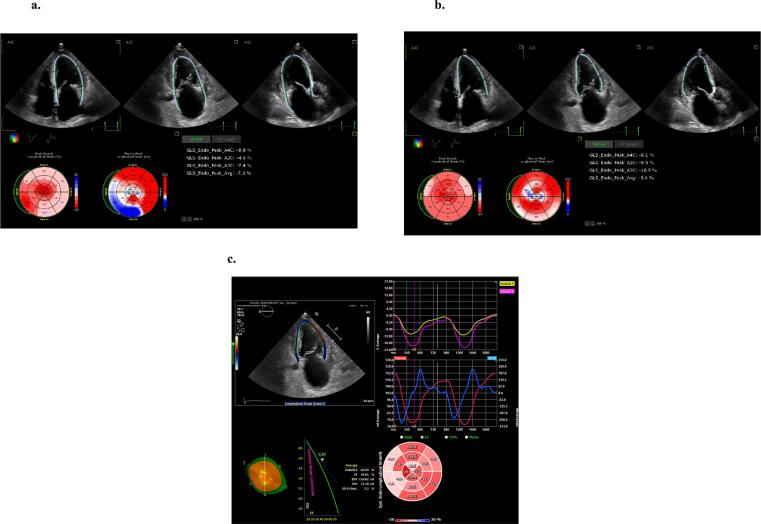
Representative case of full-automatic (a), semi-automatic (b) and manual (c) assessed LS. This was representative case of poor automatic assessment by LS. Automated trace lines were not drawn along the true endocardial border (a). Therefore, investigator manually corrected borders for semi-automatic assessment (b).2.Semi-automatic assessment: after the automatic calculation of LS, the investigator manually corrected borders (Fig. 1-b).3.Manual assessment: LS was assessed using two-dimensional cardiac performance analysis (2D-CPA): a manual measurement package (TomTec-Arena, TomTec Imaging Systems, Unterscheleis-sheim, Germany) using the defined apical 2-, 3-, and 4-chamber views for measurement. Three sampling points were manually identified at the septal and lateral mitral annulus and at the apical endocardium, and the endocardial borders were traced semi-automatically at the end of systole. After tracing the LV endocardial border, the dedicated software automatically tracked the myocardium throughout the cardiac cycle. All tracking was reviewed and manually corrected if needed (Fig. 1-c).
